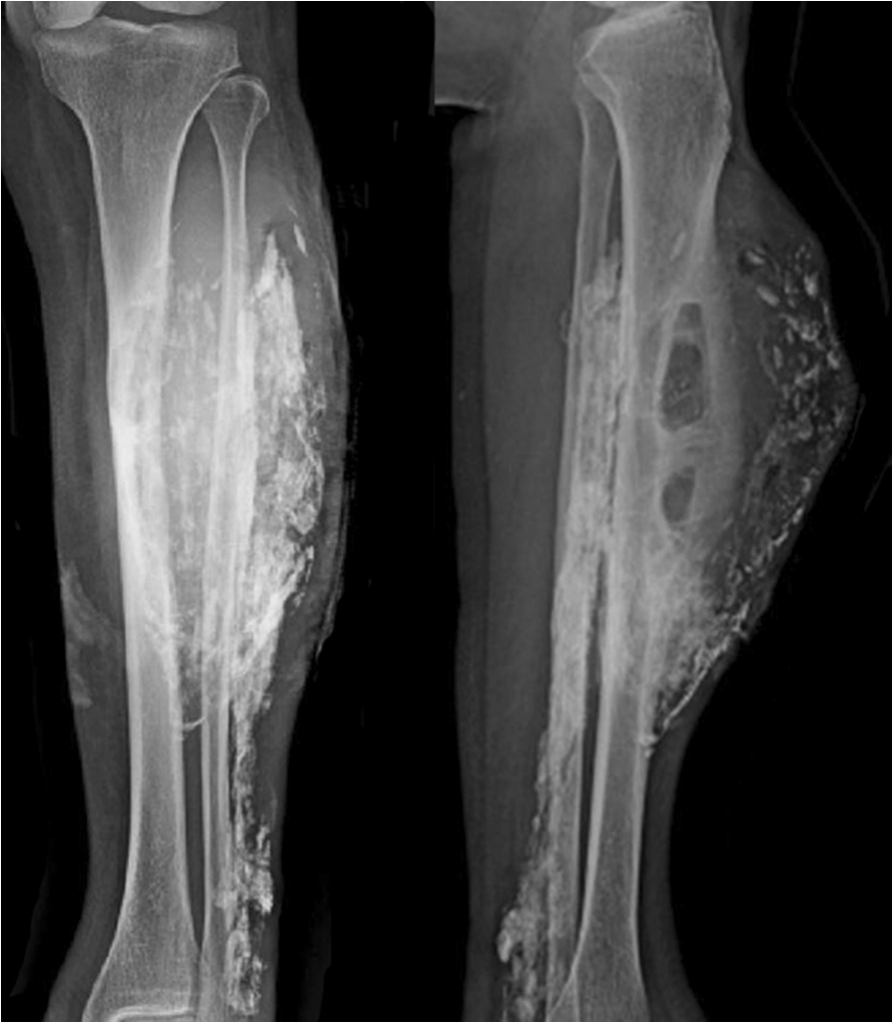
Five-year follow-up plain radiographs.
